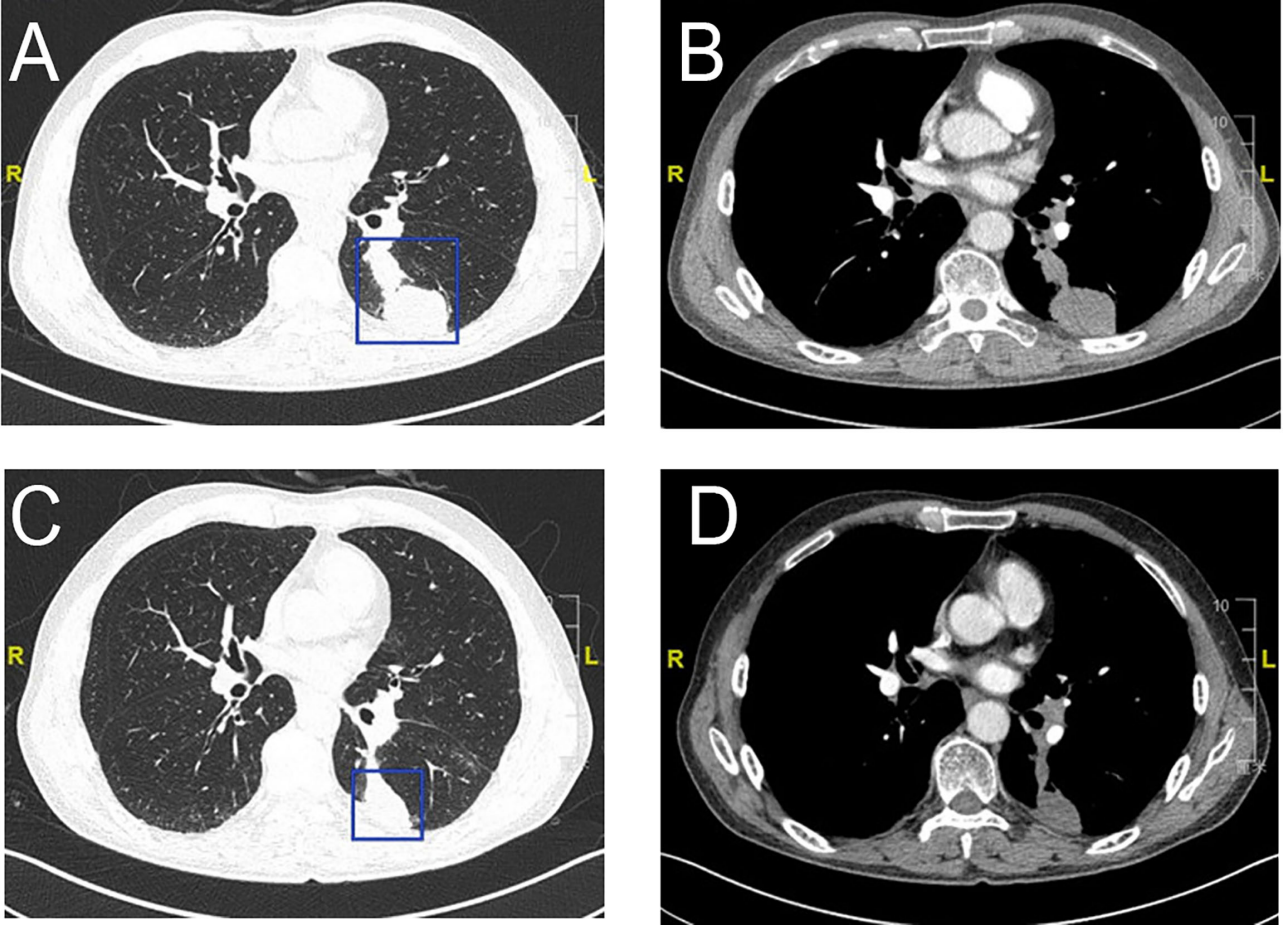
Radiologic examination of Patient 2. CT scans of the chest shows two adjacent lesions in the left lower lobe of the lung (A, B). A scan performed before surgery shows 29% shrinkage (C, D).
